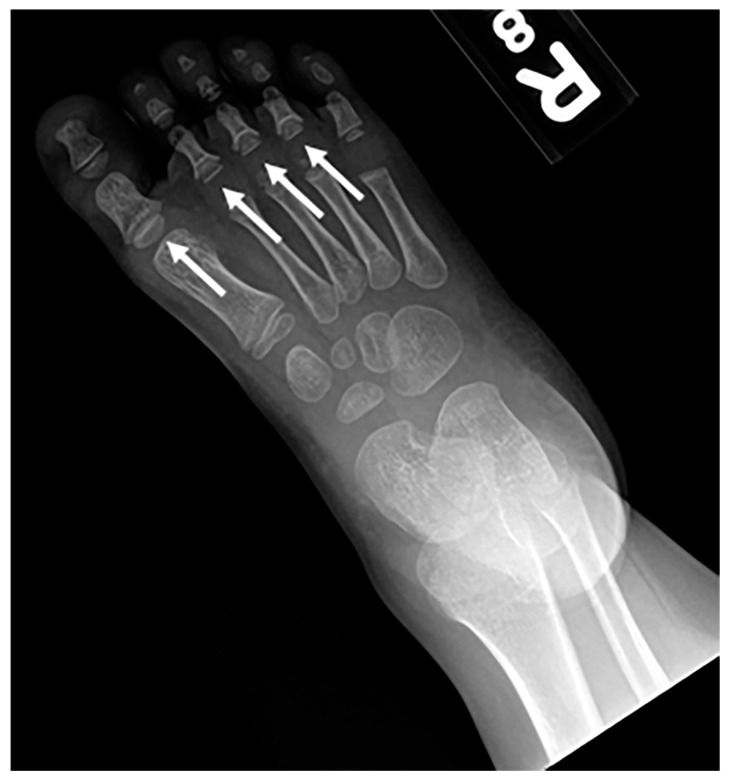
Frontal radiography of the right foot in a patient with confirmed Langer-Giedion syndrome show multiple cone-shaped epiphysis of the proximal phalanges (arrows).
